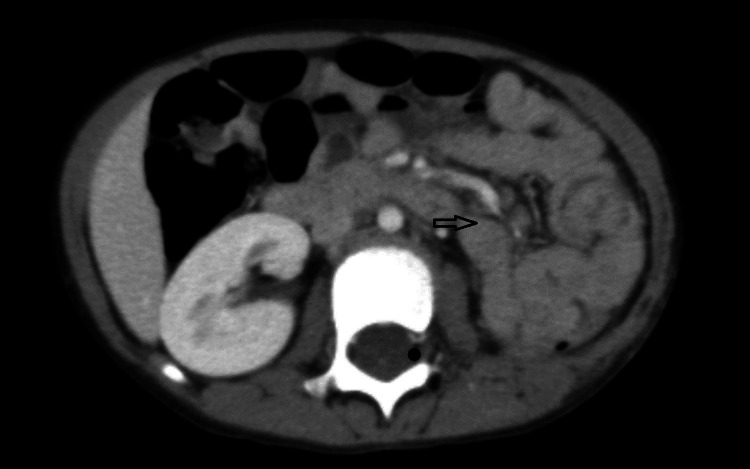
Axial CT image (21-08-2024) showing satisfactory postsurgical changes of left nephrectomy with no evidence of local recurrence. CT, computed tomography.
